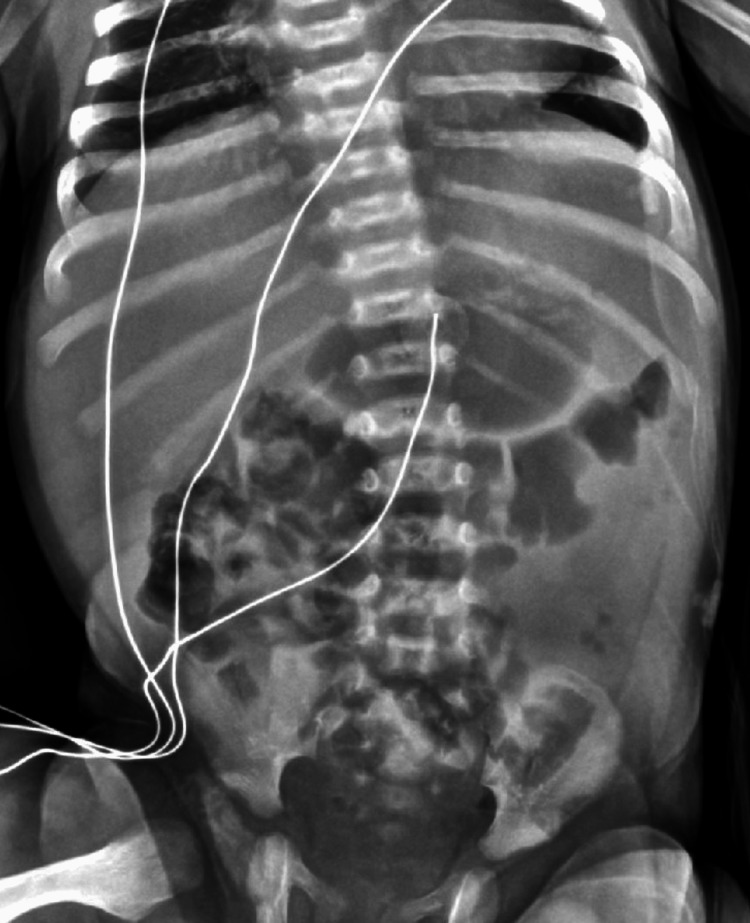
Final X-ray showing normal intestines and complete resolution of the abnormalities.
